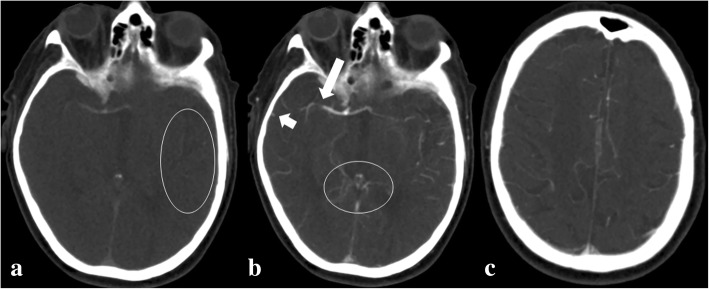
A 73-year old woman presented with sudden onset of left hemiplegia. In addition to non-contrast CT brain and CT angiography of the craniocervical arteries, CT perfusion was performed because of the uncertainty of time of symptom onset. The presented source images are images at consecutive time intervals. Source image at early arterial phase (a) shows discrete contrast filling into temporal M2 and proximal M3 branches at the normal left side (encircled). At the right side, there is only filling of the proximal middle cerebral artery with slight contrast penetration past the thrombus, but no contrast at the level of more distal M2 segment or proximal M3 segments. Source image in the later arterial phase (b, 4.5 s after a) shows almost symmetrical filling of distal arterial branches of both hemispheres. The short occlusion at distal M1 segment (long arrow) is better appreciated. The central cerebral veins are symmetrically opacified (encircled). There is already filling of the right superficial middle cerebral vein (short arrow), but persisting asymmetry in opacification of the cortical veins is observed. In late venous phase (c, 6 s after a), source image centered at a more cranial level shows symmetrical cortical vein opacification. Because of deemed adequate collateral vascular status and large penumbra/infarct mismatch at perfusion imaging (not shown), subsequently, the patient underwent successful retrieval of a small thrombus. Almost immediately after the procedure, a good clinical recovery occurred with uneventful post EVT clinical course
